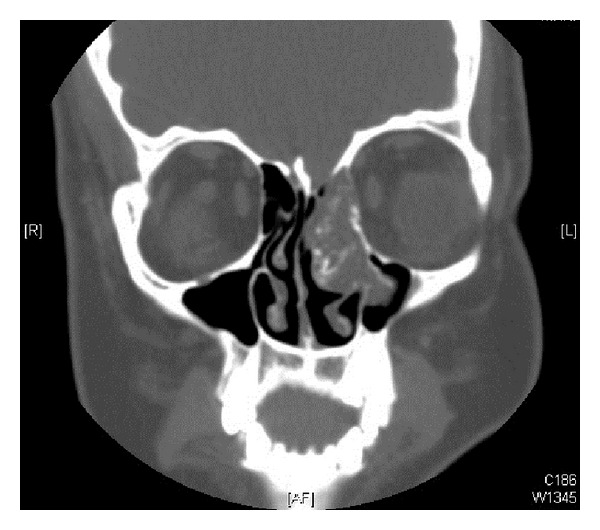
CT sinus demonstrating indolent mass with stippled calcifications centered at left middle turbinate.
